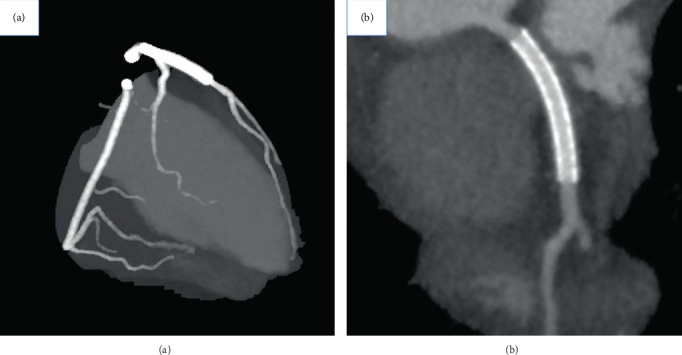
Coronary CT angiography performed 12 months after percutaneous coronary intervention. (a) Follow-up coronary CT angiography revealed no atherosclerotic stenosis in both the right and left coronary arteries. (b) There were no clear findings of in-stent restenosis or incomplete stent apposition. CT: computed tomography.
